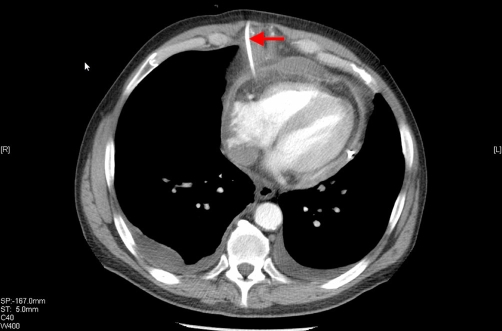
CT scan. Successful siting of the pericardial drain was seen on CT with consequent resolution of the pericardial effusion.
